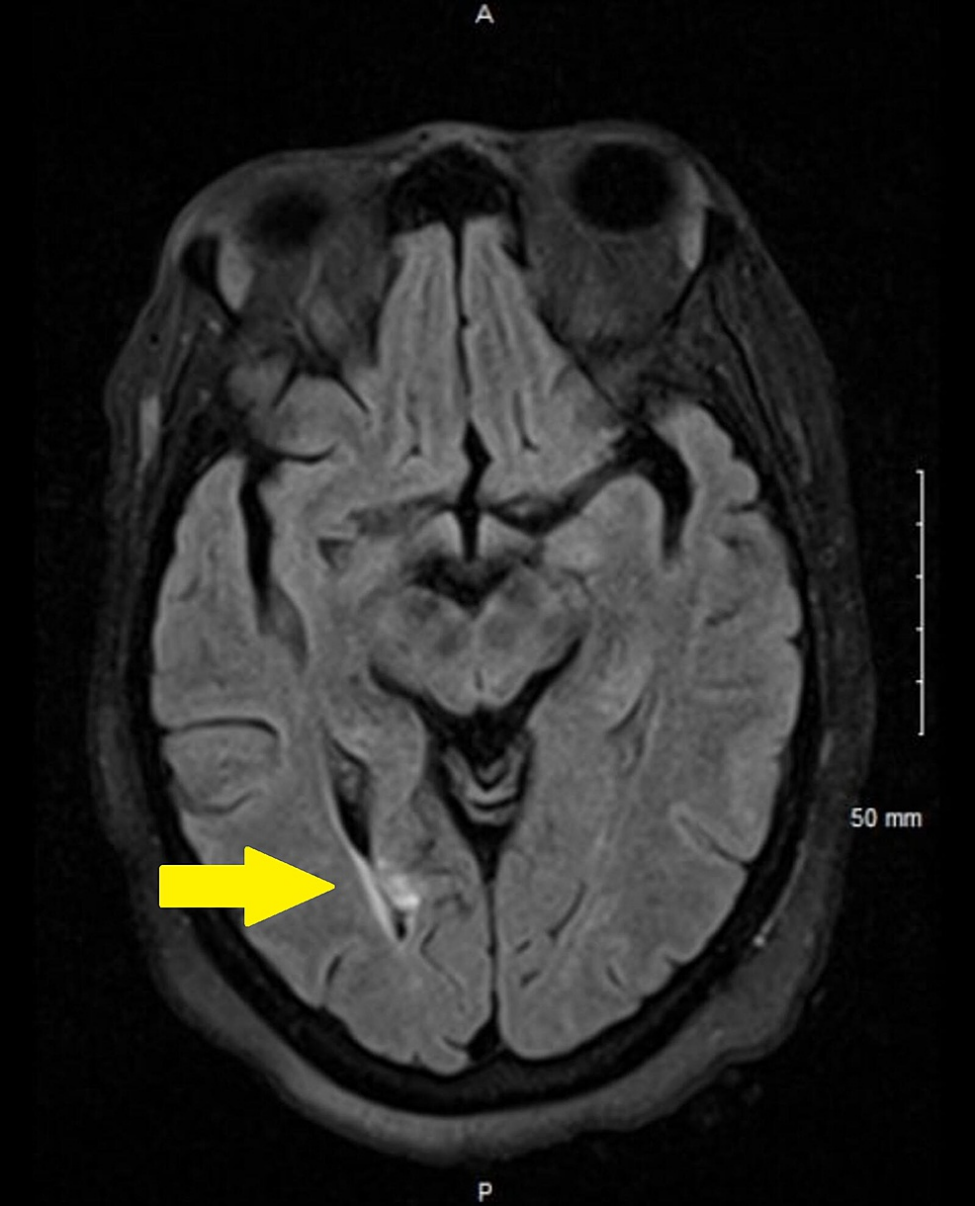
MRI without contrast of the brain (T2-FLAIR) showed acute cortical infarct which appeared as an area of increased brightness (hyperintense) in the right parieto-occipital region. T2-FLAIR, T2-weighted fluid-attenuated inversion recovery
